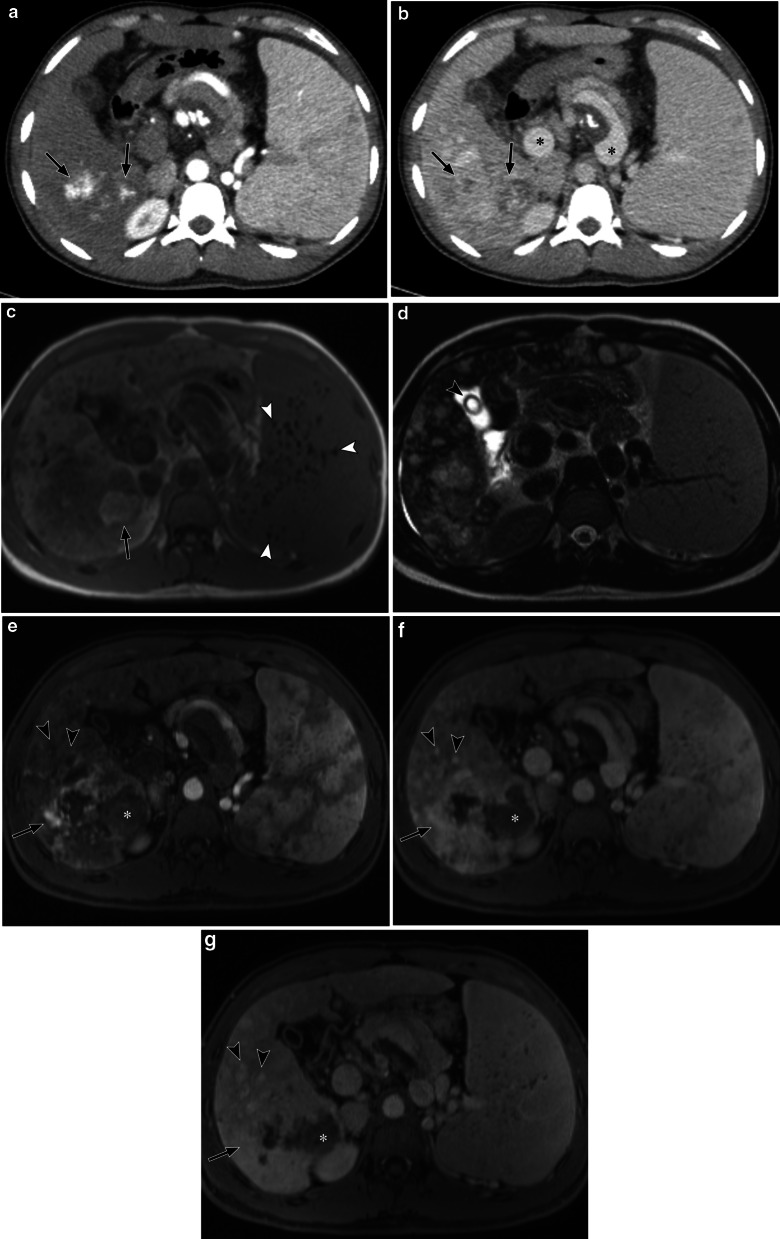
30-year-old man with primary hepatic angiosarcoma and longstanding portal hypertension from microvascular veno-occlusive disease. a, b Axial contrast-enhanced CT images of the abdomen show a dominant mass in the posterior right hepatic lobe with smaller multifocal nodules. a Arterial phase CT image shows central foci of avid enhancement within the dominant lesion (arrows). b Portal venous phase CT shows progressive heterogeneous enhancement (arrows). There are features of portal hypertension with distension of the portal and hepatic veins (asterisks) and splenomegaly. c Axial T1-weighted in-phase image shows multifocal predominantly low signal intensity lesions compared to the adjacent liver parenchyma. There is a focus of high signal intensity within the large lesion posteriorly suggestive of haemorrhage (arrow). Small low T1 signal nodules within the enlarged spleen represent haemosiderin deposition related to portal hypertension (arrowheads). d Axial T2-weighted image shows multifocal heterogeneous hepatic lesions which are of predominantly high signal intensity. There is an incidental calculus within the gallbladder (arrowhead). e–g Axial T1-weighted dynamic contrast-enhanced MR images of the liver. Arterial (e), portal venous (f) and delayed-phase (g) images show peripheral nodular enhancement of the dominant lesion with progressive enhancement (arrow) and a persistent area of non-enhancement (asterisk). Smaller nodules elsewhere in the liver also show progressive enhancement but appear relatively homogeneous compared to the dominant lesion (arrowheads)
